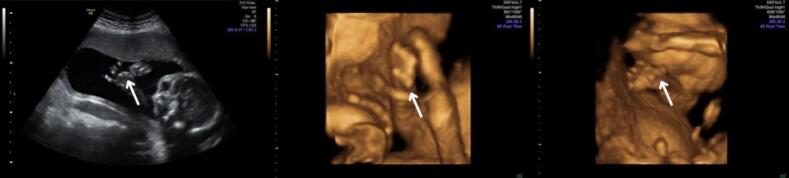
Two-dimensional and three-dimensional ultrasound images showing the curvature of the thumb indicating clinodactyly.
